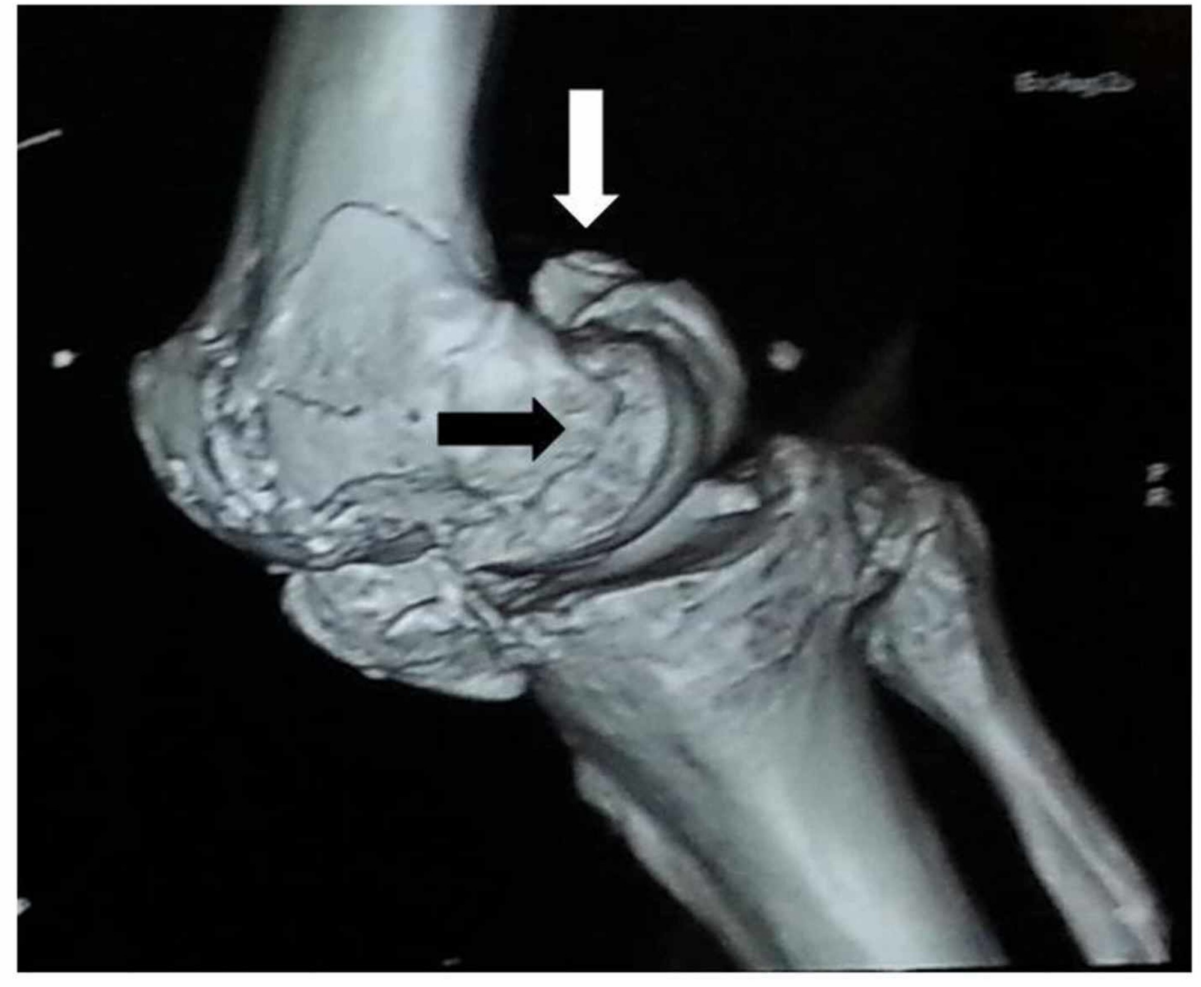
3-D computed tomography showing bicondylar Hoffa’s fracture: medial condyle (black arrow) and lateral condyle fracture (white arrow)
